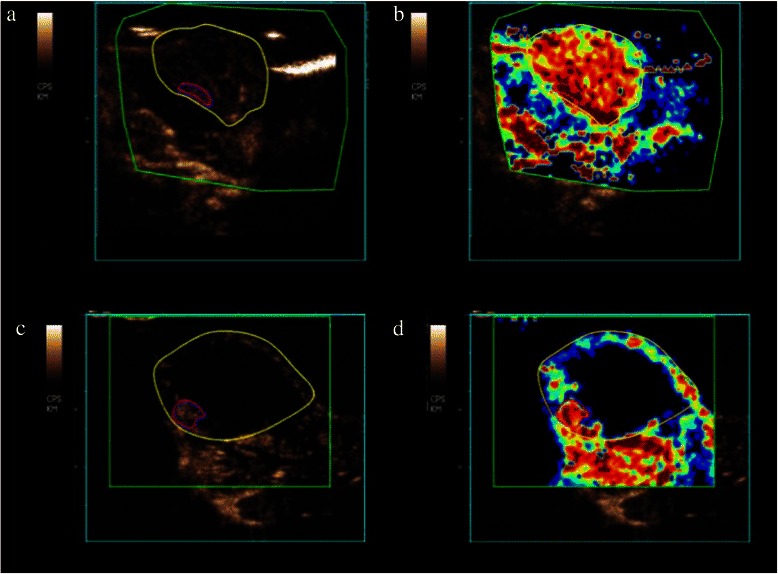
The contrast-enhanced ultrasound image shows a significant reduction of the vascularization between the baseline (a) and follow-up (c) scan. Although the tumor grew in size during treatment, must of the tumor is necrotic. The blood volume parameter maps show a corresponding decrease of the relative Blood Volume (rBV) within the remaining hypervascular tumor betwenn the baseline (b) and follow-up (d) scan.
